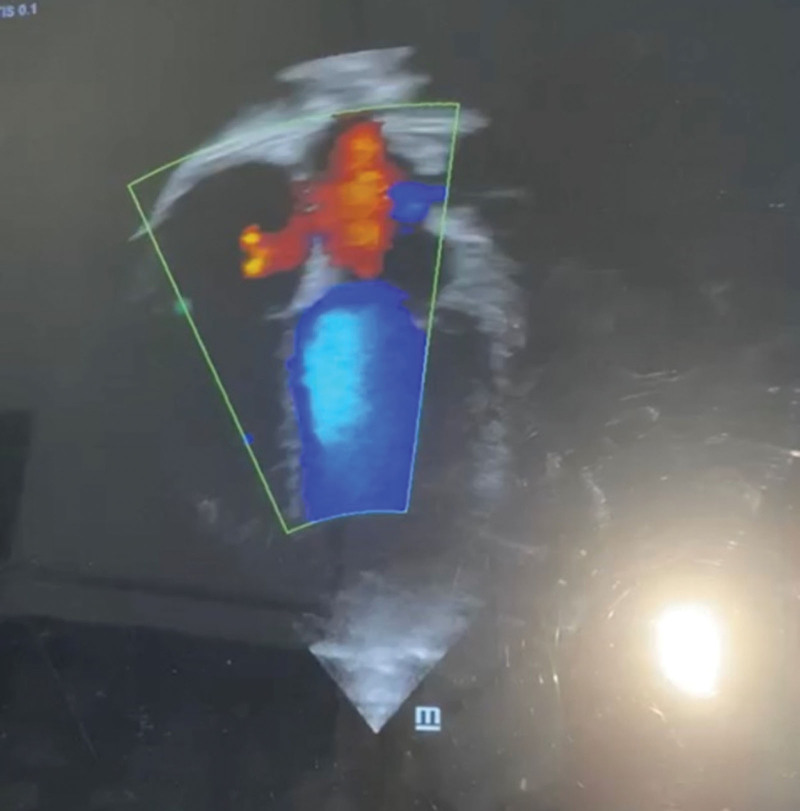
Echocardiographic image showing ostium secundum atrial septal defect.
